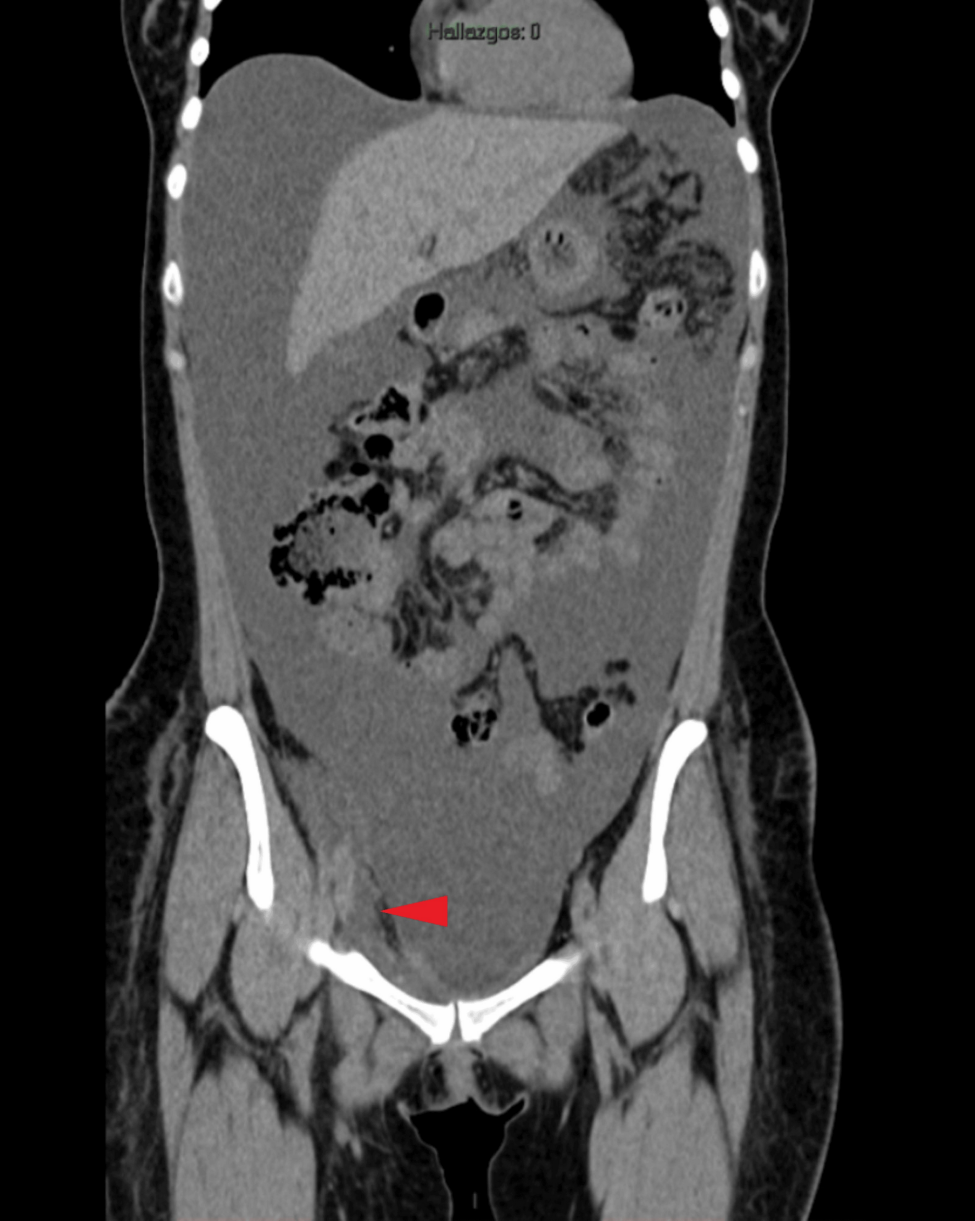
CT scan showing peritoneal cavity with massive ascites and the fluid collection in the right groin (red arrowhead). CT: Computed tomography
